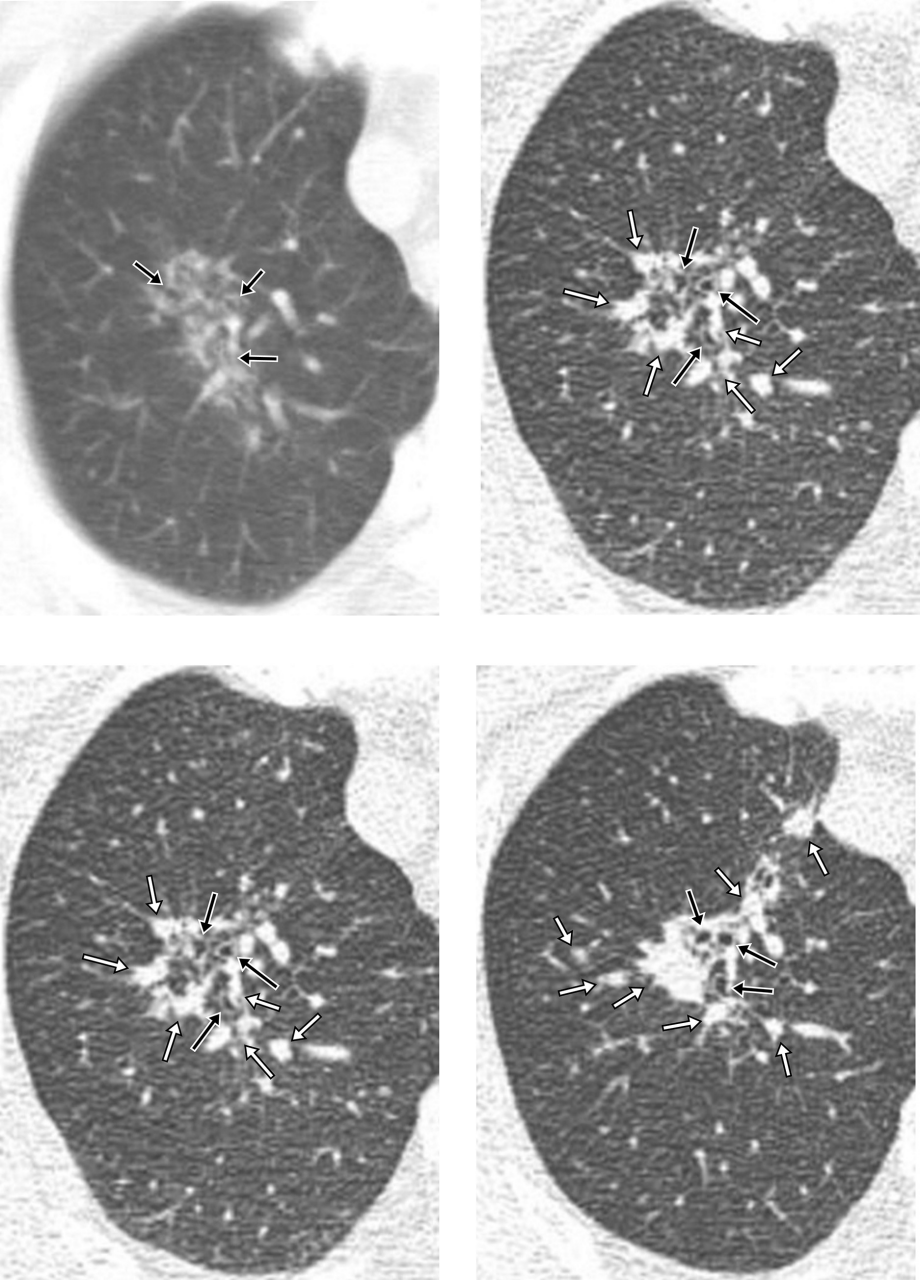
Active pulmonary tuberculosis with predominant radiologic findings of Clustered Micronodules (CMs) in a 68-year-old male patient over the course of 76 months. (A), A pre-diagnostic CT image taken 76 months before the diagnostic CT scan shows a focal aggregation of micronodules around the small airway with wall thickening (*arrow*) that cannot be explained by centrilobular or miliary distribution in the apical segment of the right upper lobe (CT score 3). (B), (C) and (D), Three serial CT images obtained 48 months, 13 months, and 0 month (diagnostic CT) before the diagnosis of active pulmonary TB. The CMs distributed along the bronchiolectasis (*black arrow*) were stable during follow-up until 48 months before the diagnosis, and then gradually progressed to consolidations and nodules (*white arrow*) starting 13 months before the diagnosis (CT score 8).
